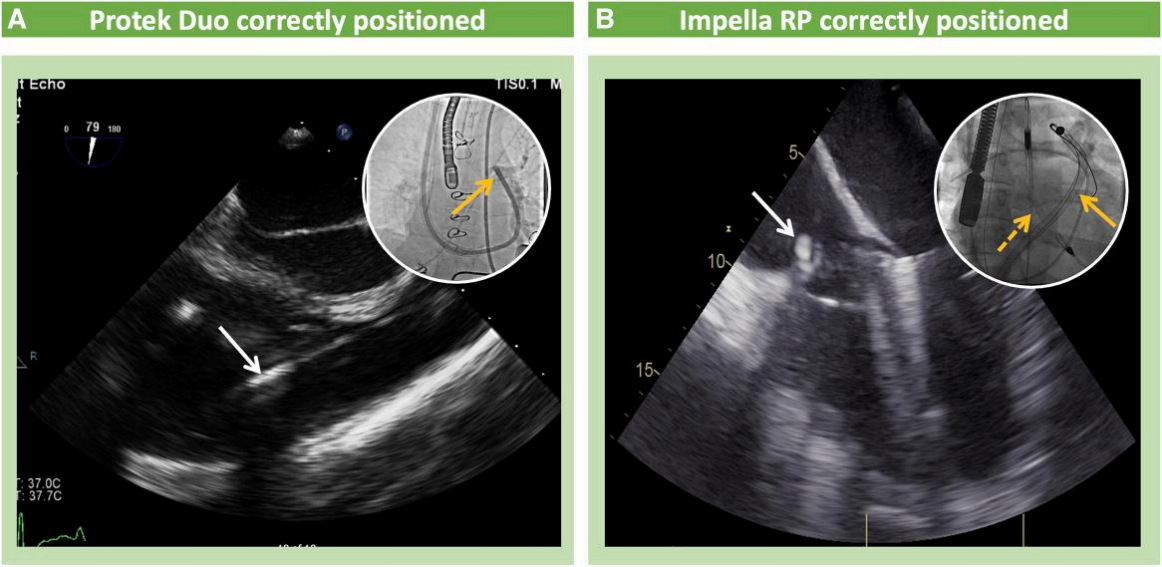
Echocardiographic guided positioning of Impella RP and ProtekDuo. (*A*) TOE assessment of the ProtekDuo cannula. (*A*) Modified mid-oesophageal RV inflow–outflow view showing the device (white arrow) extending into the RV and across the pulmonary valve into the PA. Fluoroscopy anteroposterior view of the same device (white-framed circle) seen entering the RA via the SVC with the device outflow in the PA (yellow arrow). A PA catheter (white arrow) from the right internal jugular vein is also seen. (*B*) TOE assessment of the Impella RP device. Mid-oesophageal four-chamber view showing the device’s body angle (white arrow) traversing the TV valve. (*B*) Fluoroscopy anteroposterior view of the same device shows Impella RP device (yellow arrow) positioned in the RV from the IVC and the Impella CP device (yellow dashed arrow) (BiPELLA configuration).
